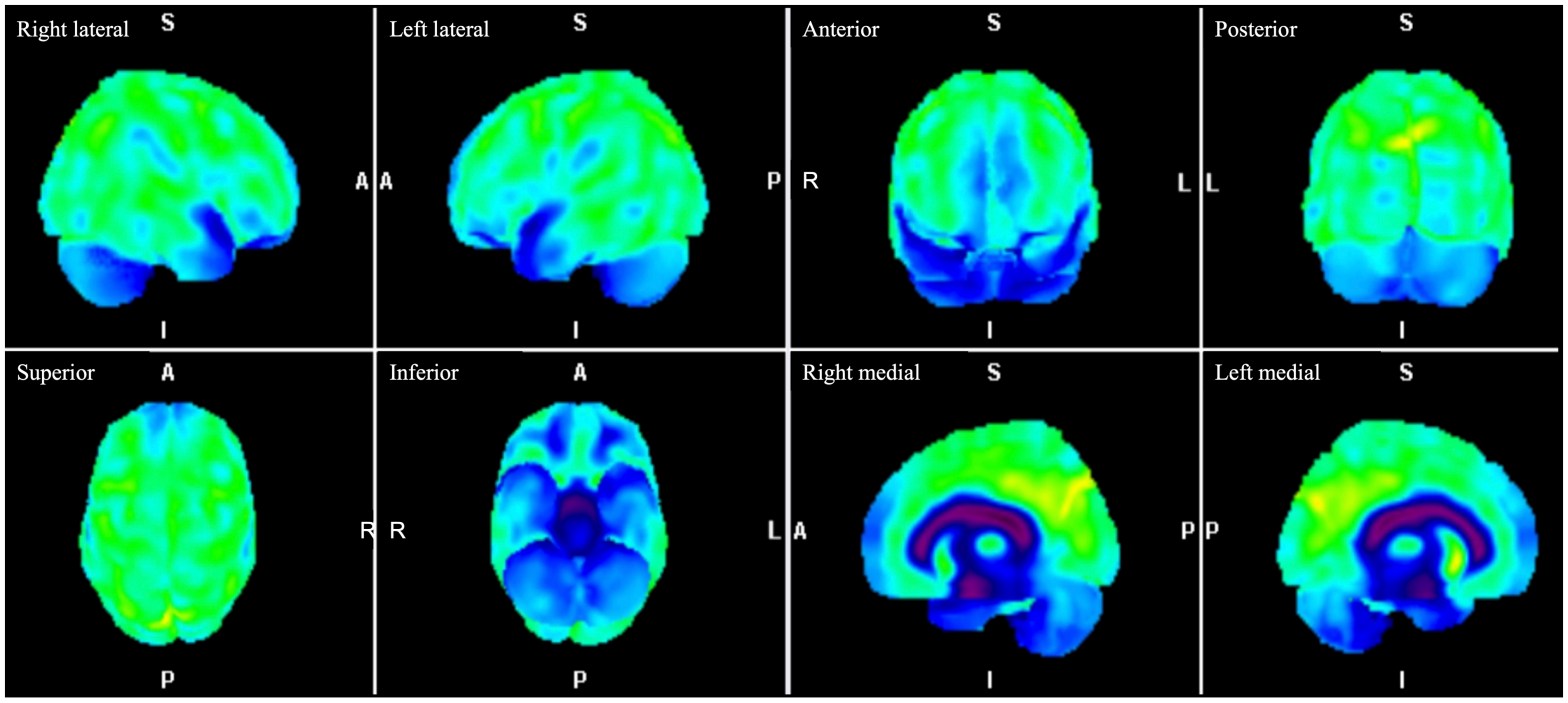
Brain ^18^F-FDG PET images. Three-dimensional stereotactic surface projection images of the right lateral, left lateral, anterior, posterior, superior, inferior, right medial and left medial projections demonstrate hypometabolism in the frontal lobes, with greater hypometabolism toward the superior orbital gyrus and inferior opercular, triangular, and opercular gyri, with less involvement of the temporal lobe. ^18^F-FDG PET, fluorine-18 fluorodeoxyglucose positron emission tomography.
